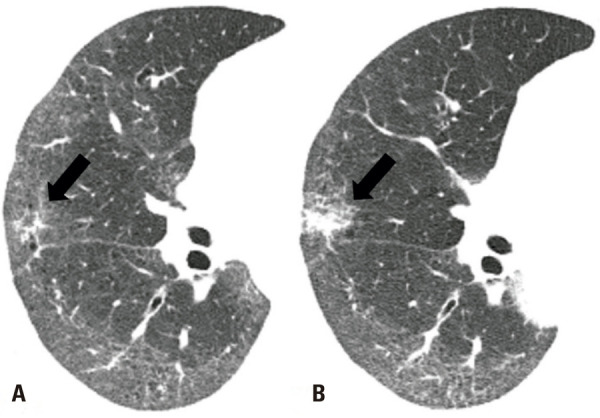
A 69-year-old woman with a fibrotic NSIP pattern owing to systemic sclerosis. Axial chest computed tomography images show a subtle irregular opacity in the right middle lobe (arrow in A), which increased in size at the 1-year follow-up (arrow in B) and was diagnosed as squamous cell carcinoma
